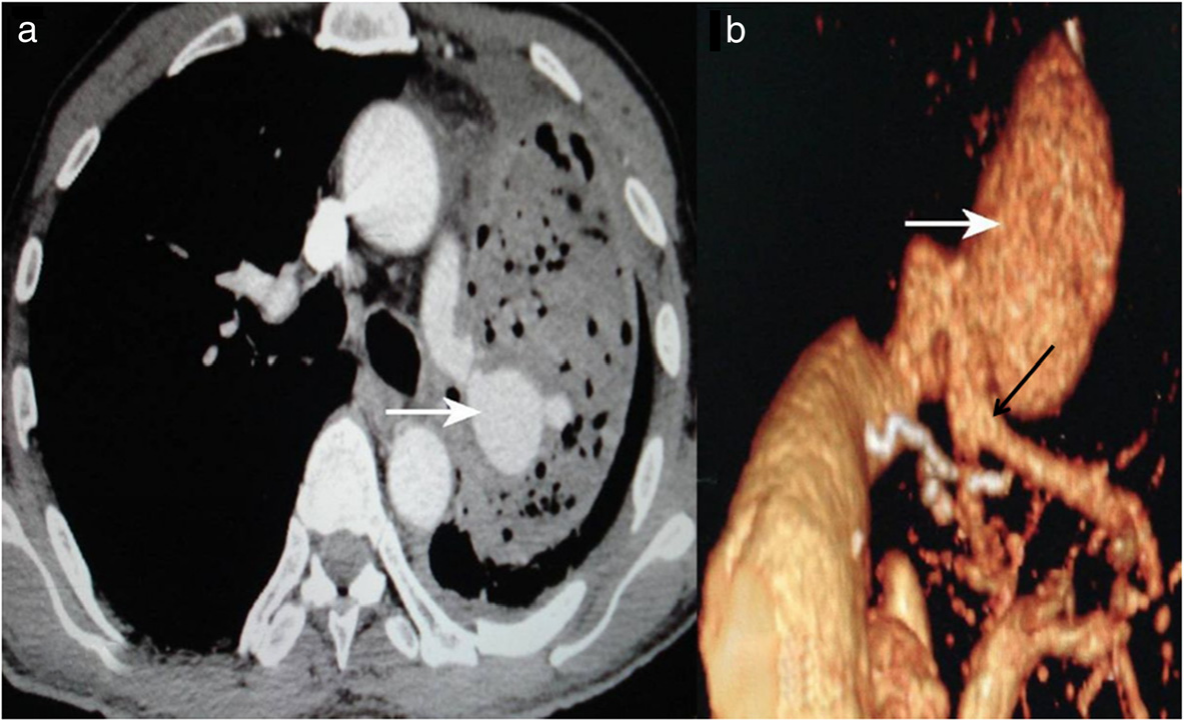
Three-dimensional (3-D) radiography confirmed a pseudoaneurysm feeding from the dorsal segment branch of the left lower lobe with apparent proximal arterial enlargement. The pseudoaneurysm (white arrow) was 3.2 × 2.8 cm in size and surrounded by infected thrombus, pleural effusion, and lung tissue (a). The pseudoaneurysm was also apparently huge compared to the feeding pulmonary artery under 3-D imaging (b, white arrow). The angioplasty lesion was indicated by a black arrow (b).
